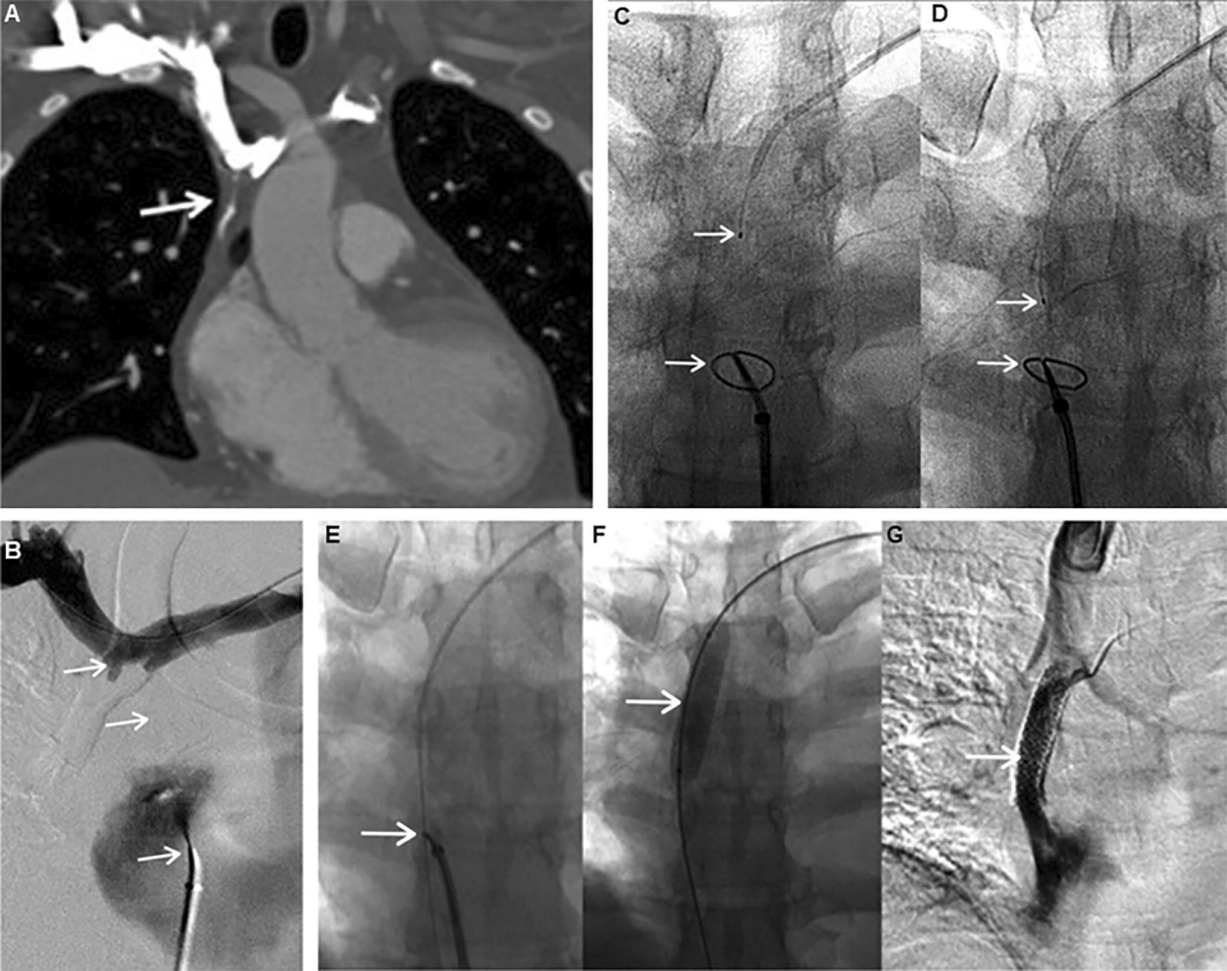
Sniper technique for recanalization of a long segment superior vena cava occlusion. A Coronal CTA of the chest demonstrates a long segment occlusion of the superior vena cava, which extends from the brachiocephalic confluence to the right atrium. Faint linear contrast opacification is noted extending from the brachiocephalic confluence, which was the sequela of a prior recanalization attempt (white arrow); B Central venogram performed simultaneously from above (top white arrow) and below (bottom arrow) the occluded SVC (middle white arrow); C, D The radiofrequency wire (upper white arrows) and the target snare (bottom arrows) in alignment with incremental advancement toward the snare, ensuring proper trajectory with multiple fluoroscopic projections; E–G Successful traversal of the radiofrequency wire through the occlusion, which was snared out (left white arrow) for through-and-through access for subsequent angioplasty (middle white arrow) and stenting (right white arrow) of the SVC, which demonstrated patent stent without extravasation on final venogram [8]
